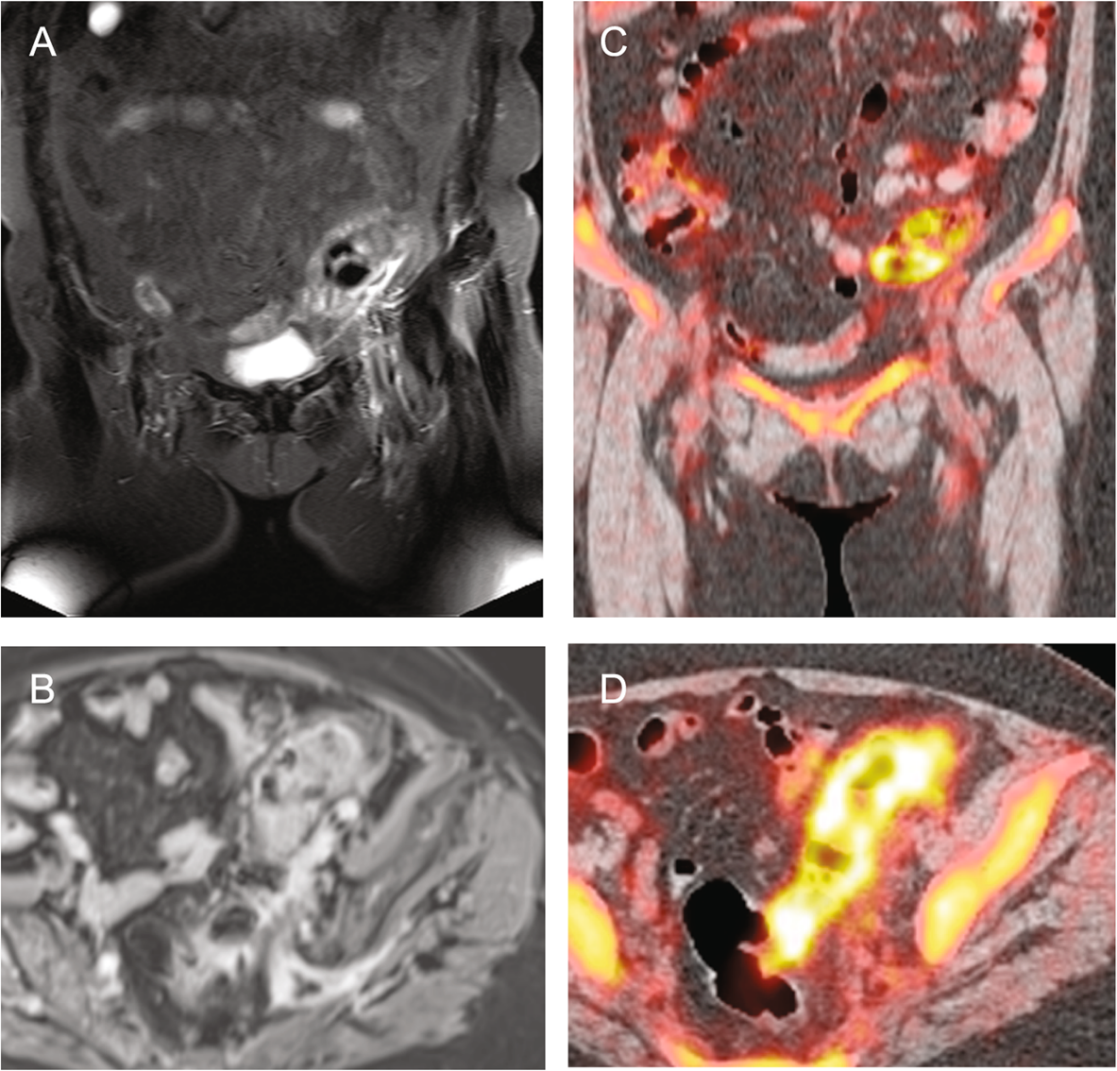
76-year-old patient with FUO suffering from occult sigmoid diverticulitis who underwent both wb-MRI and PET-CT. Coronal T2-STIR a and axial contrast enhanced T1-VIBE b images reveal sigmoid wall edema and perifocal fat-stranding in accordance with sigmoid diverticulitis. Coronal and axial FDG-PET-CT c, d display elevated tracer uptake of the sigmoid as sign of acute inflammation
